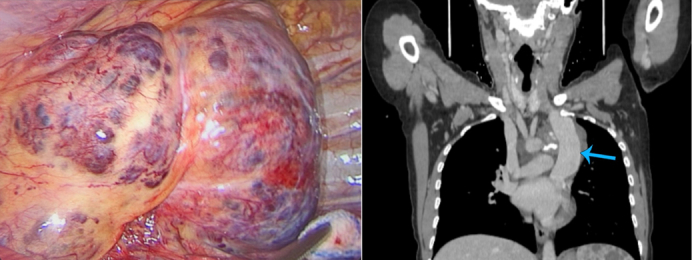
The highly vascular cavernous hemangioma and CT imaging of the dilated left PSVC.
